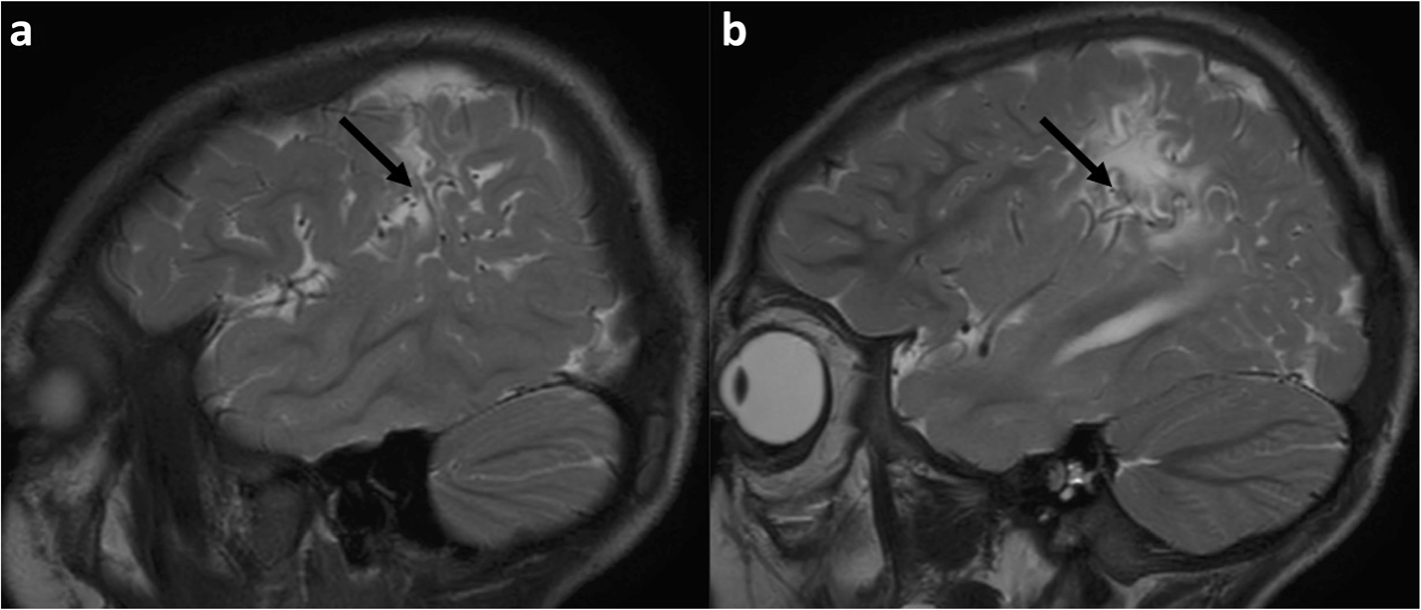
a, b Isolated peri-Sylvian watershed involvement in a 12-year-old girl. Sequential T2-weighted MRI slices in the sagittal plane demonstrate atrophy of the cortex and subcortical signal abnormality with white matter volume loss, at the most superior portion of the Sylvian fissure (black arrows), which represents an ‘end-zone’ of all three major cerebral arteries and represents the most commonly involved portion of the watershed zone in patients sustaining partial-prolonged HII at term gestation
